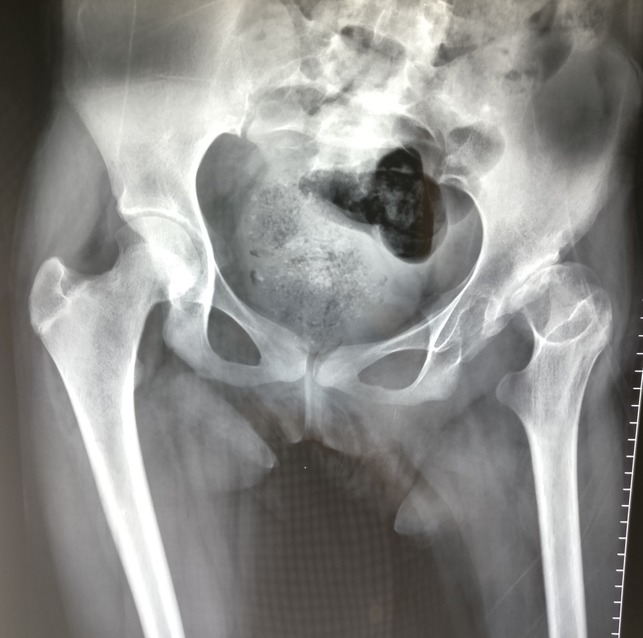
Radiograph showing the anterior-posterior view of the pelvis with both hips demonstrating a neglected dysplastic hip on the left side.
